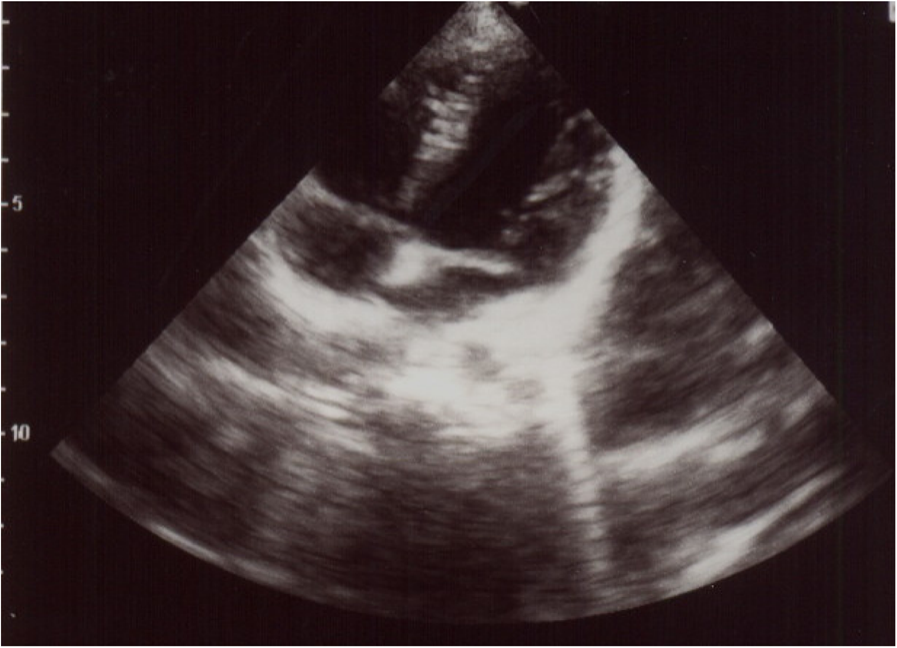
Transthoracic echocardiography revealed normal four-chamber size and function, except for a mild LVH and mild tricuspid regurgitating with an LVEF of 55%
